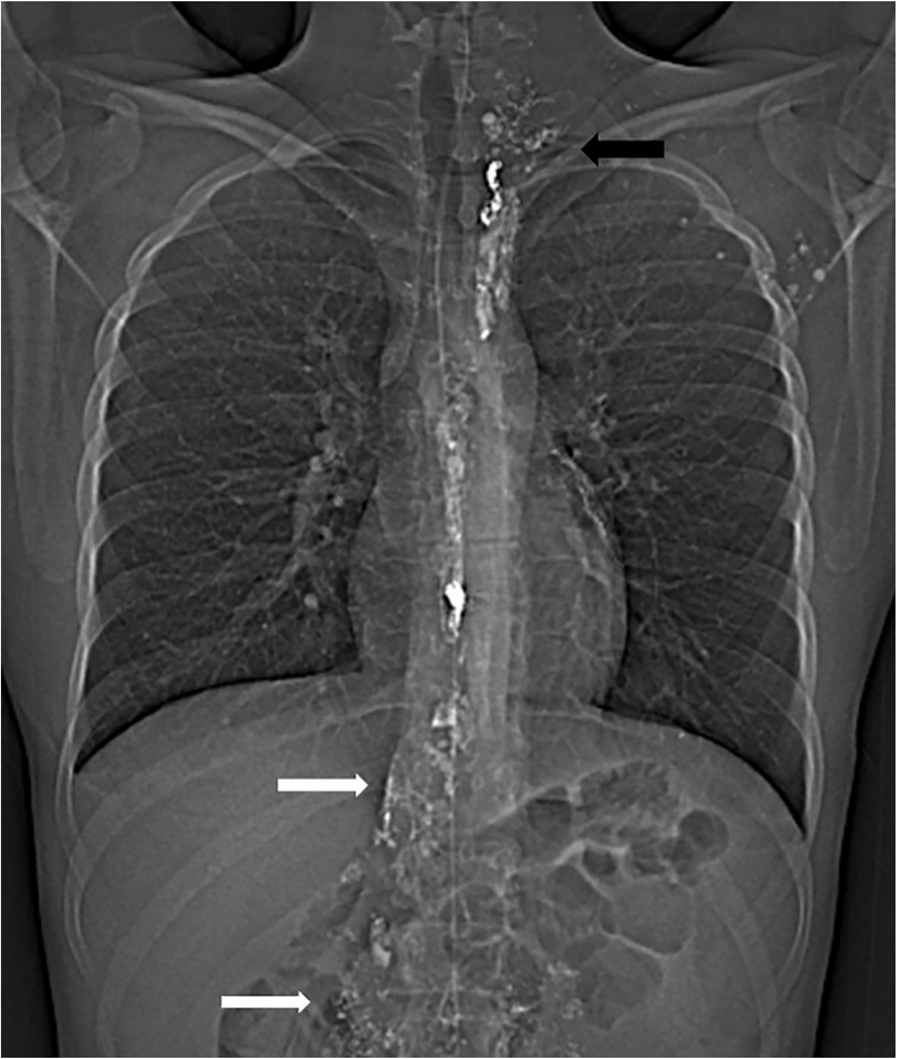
Post-procedural CT topogram demonstrating embolization coils and NBCA through the length of the thoracic duct. Ethiodol also opacifies portions of the malformation in the abdomen (white arrows) and mediastinum/left neck (black arrow) along with left axillary lymph nodes
